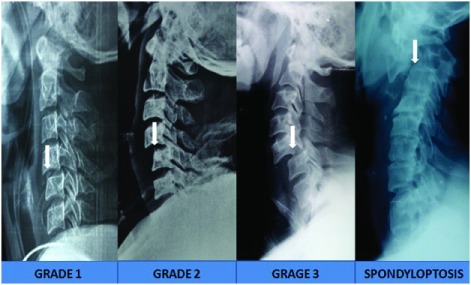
X-ray images showing various grades of traumatic subluxation.
